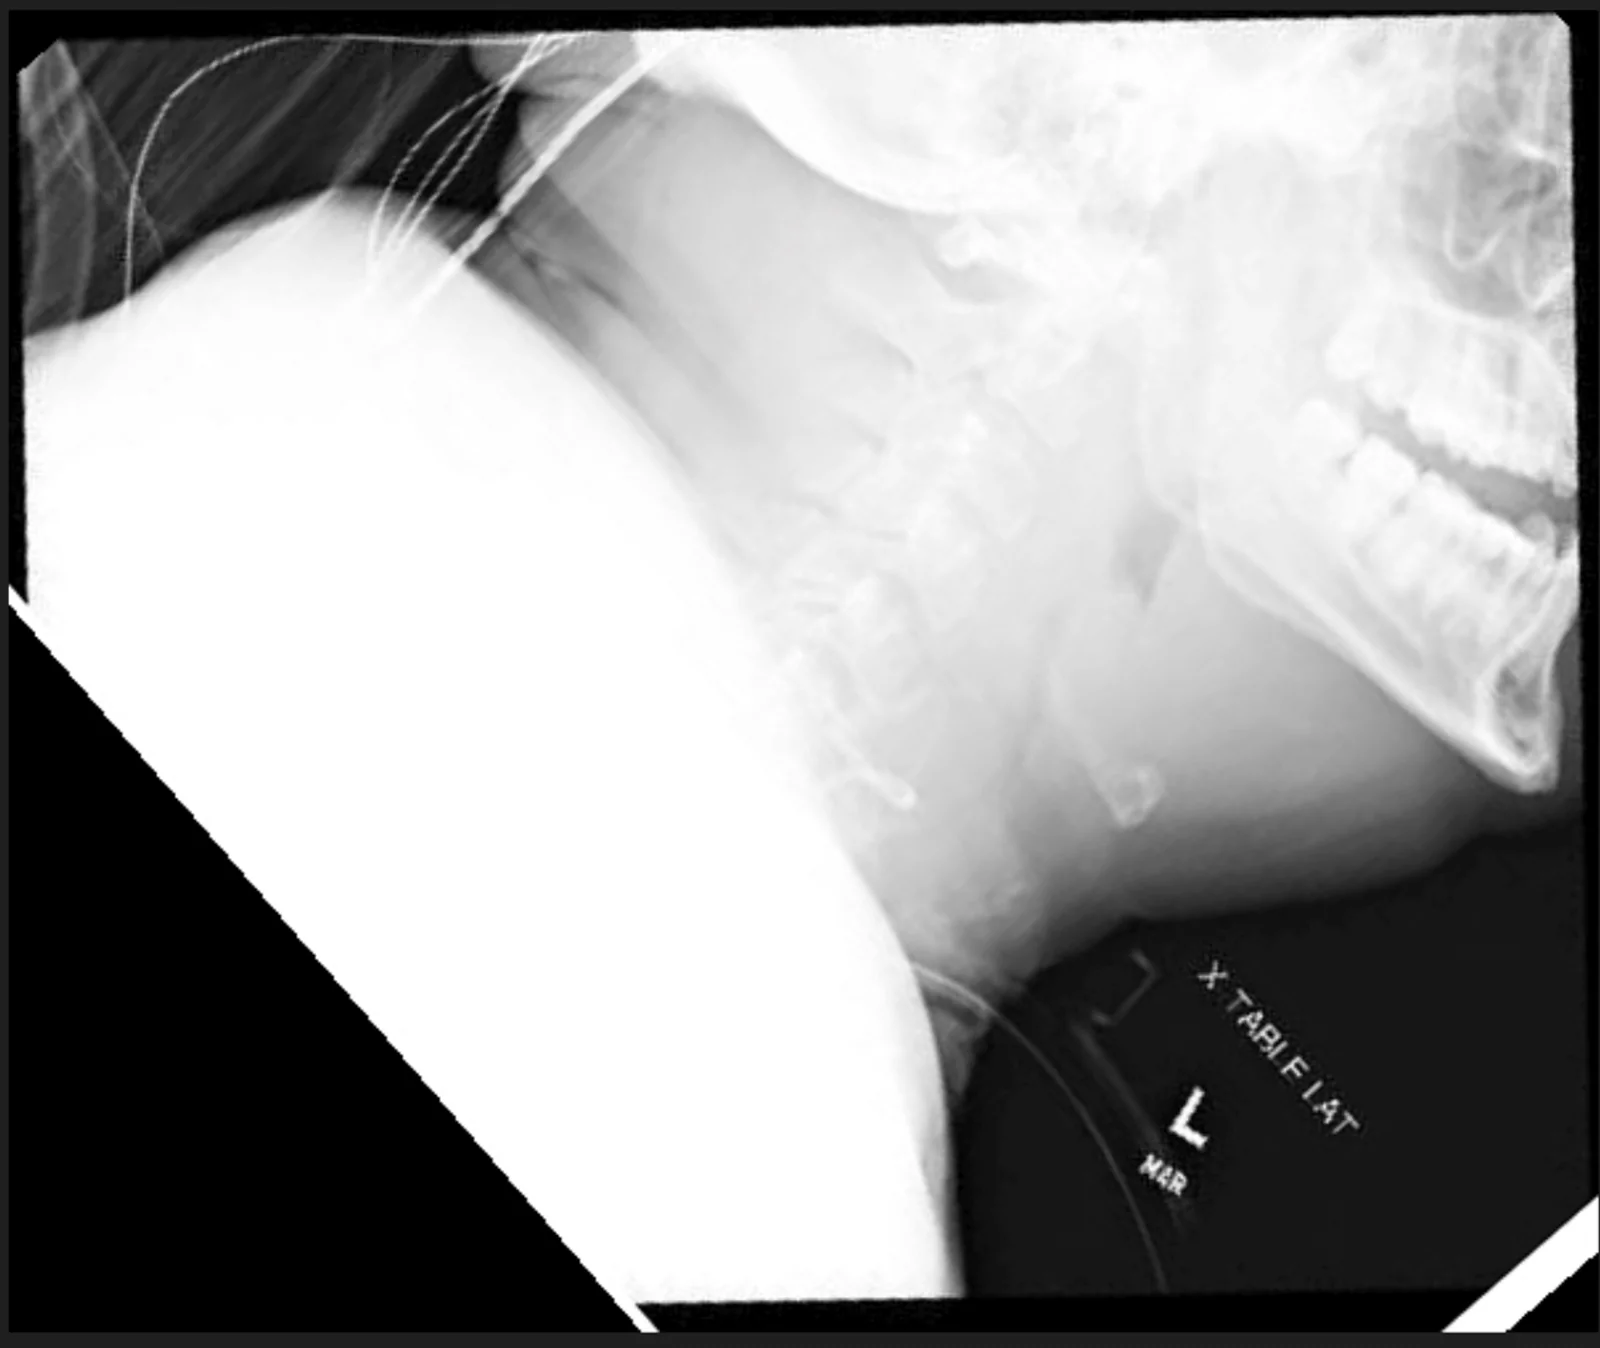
Cross-table lateral neck radiograph following cricothyroidotomy Cross-table lateral radiograph of the neck taken after emergency cricothyroidotomy, confirming the position of the cuffed tracheal tube through the cricothyroid membrane and showing the supraglottic airway still completely obstructed.
